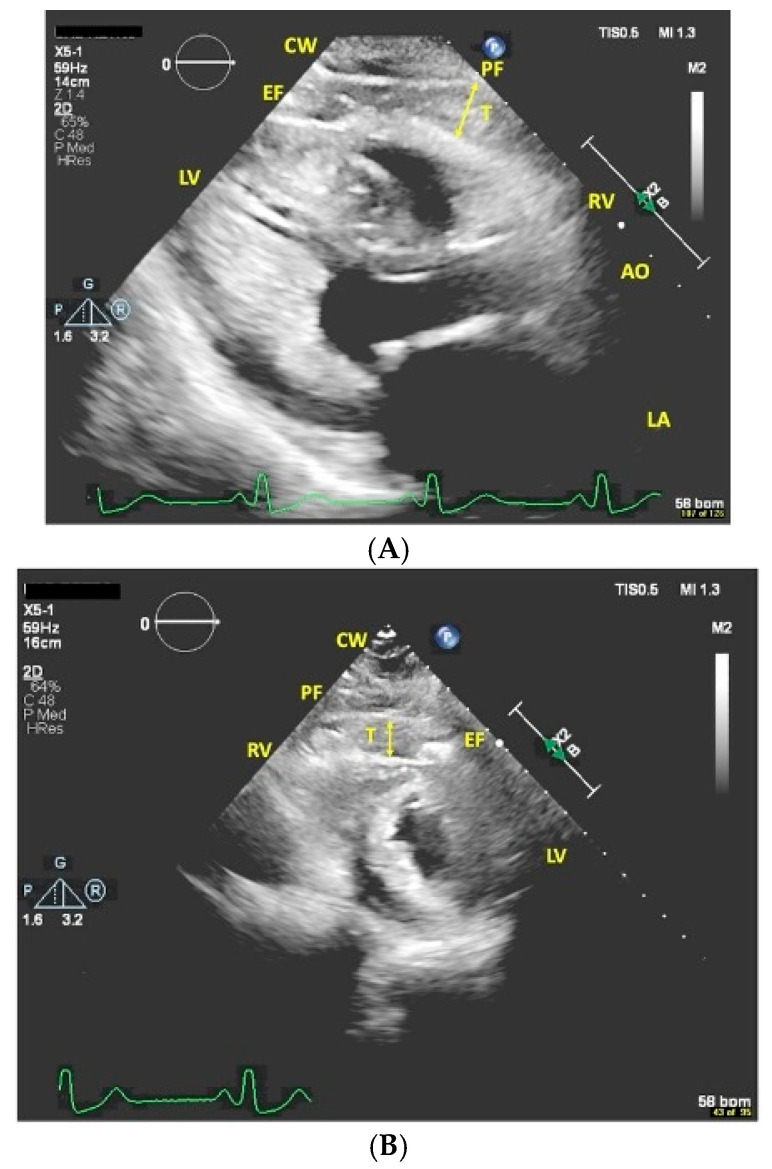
(A): Two-dimensional transthoracic echocardiographic assessment of epicardial adipose tissue (EF). Maximal thickness (T) of EF is 13 mm. Parasternal long-axis view. AO: Aorta; CW: Chest wall; LA: Left atrium; LV: Left ventricle; RV: Right ventricle; PF: Pericardial fat pad. Figure courtesy of Navin Nanda MD. (B): Two-dimensional transthoracic echocardiographic assessment of epicardial adipose tissue (EF). Maximal thickness (T) of EF is 14 mm. Parasternal short-axis view. Abbreviations as in Figure 6A. Figure courtesy of Navin Nanda MD.
